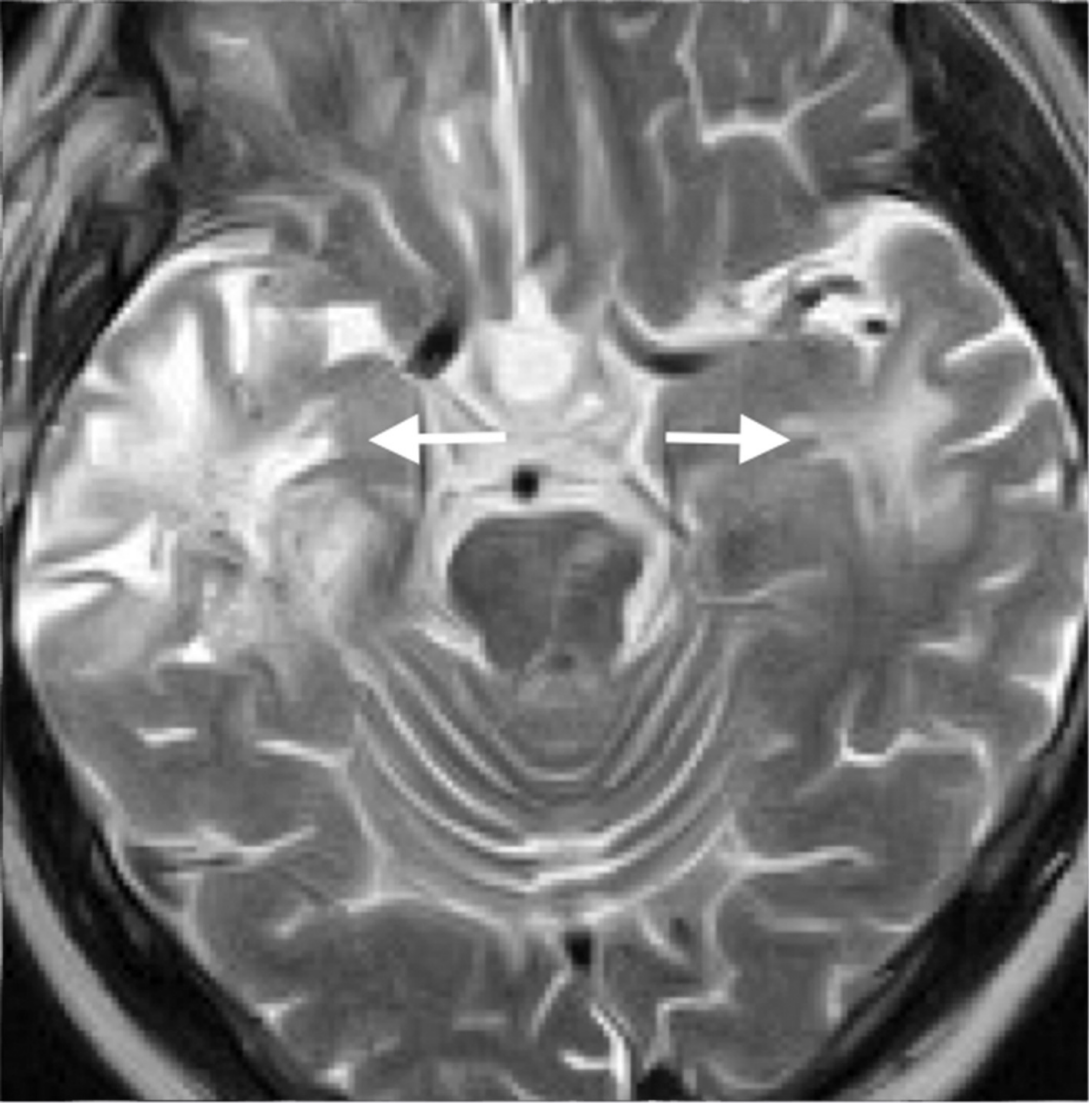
An MRI performed 1 year ago shows hyperintense areas in the bilateral temporal lobes, indicating radiation-induced change on a *T*
